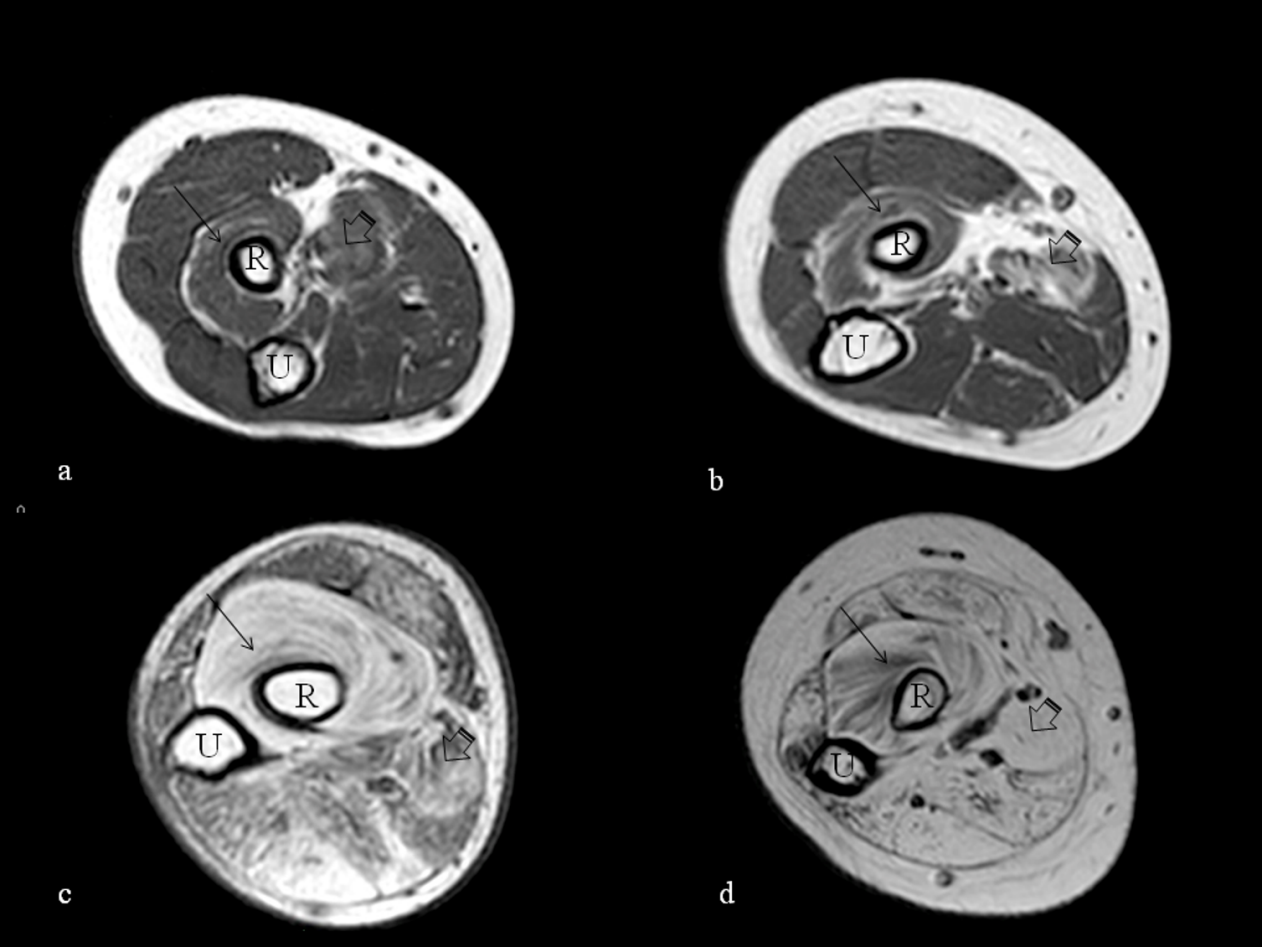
T1-weighted axial MRI of the forearm. T1-weighted axial MRI of the forearm at the level of the radial tuberosity showing increased signal suggestive of fatty replacement in the forearm muscles, especially in the supinator (arrow) and pronator teres (arrowhead). The involvement is milder in the youngest ambulant patients aged 8 and 14 (a and b respectively) and more marked in the older non-ambulant ones, both aged 17 (c and d), with severe involvement shown in image d. R (radius); U (ulna).
